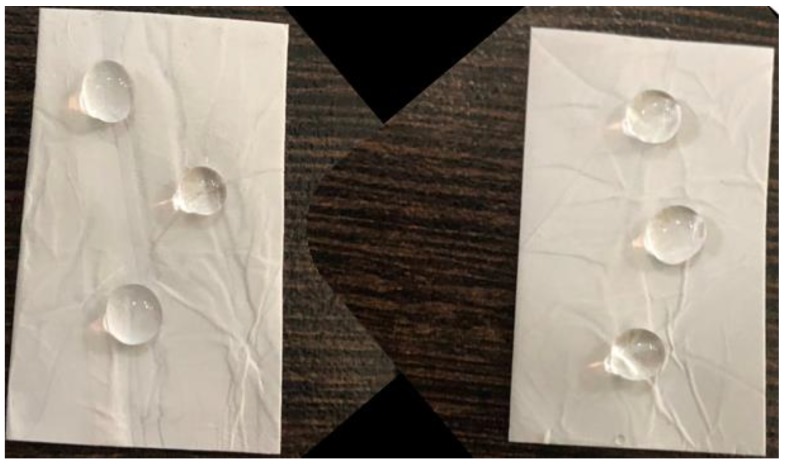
Photographs of water droplets on the PS/hBCo-O gamma-irradiated fiber surface before and after ultrasonication.
